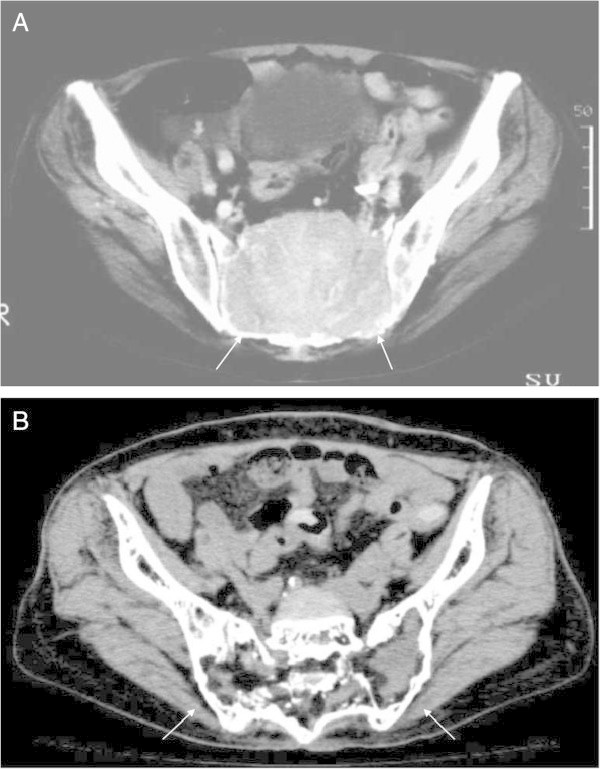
A 68-year-old female with GCT in the sacrum (Case 2). A. On CT, a large osteolytic mass occupied the sacrum (white arrows). B. On CT of same level as Figure 1A about 10 years after initial TAE, the size of the mass decreased and obvious reossification is shown (white arrows).
